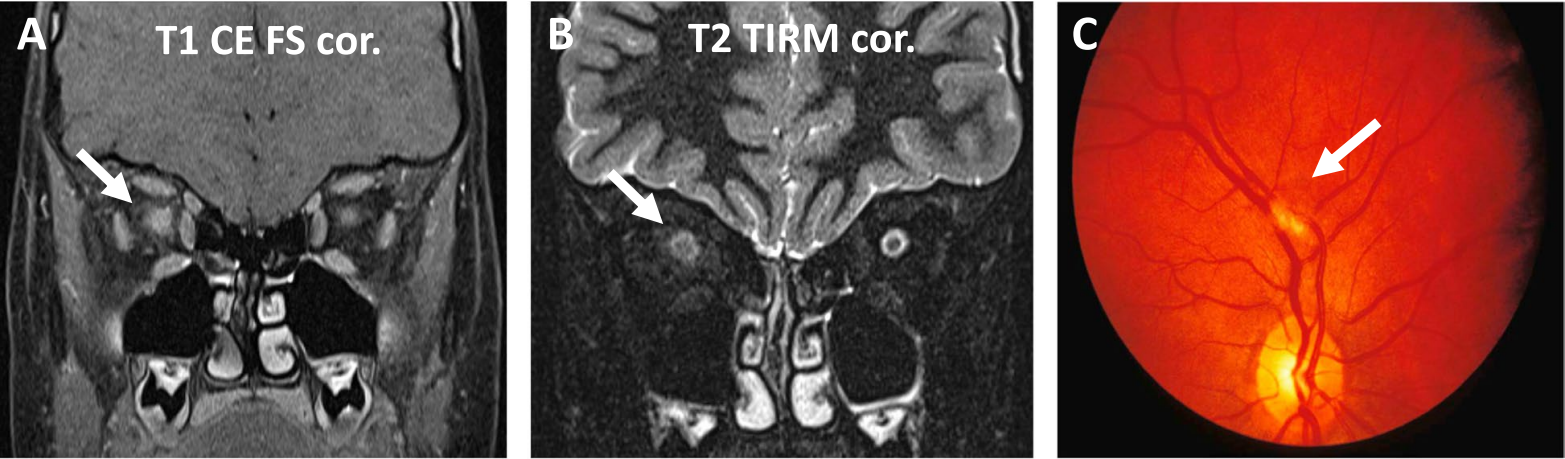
Neuromyelitis optica and retinitis in an 8-year-old jSLE patient. A Coronal MRI images showing perineural contrast enhancement of the right optic nerve in T1-weighted sequences (arrow). B Increased T2-weighted signal of the *Nervus opticus* and reduced signal of the nerve sheath (arrow) representing focal oedema of nerve and optic perineuritis. C Cotton-wool infiltrates resembling retinitis of the right eye in the same patient. The patient failed to respond to treatment with CPM and high-dose corticosteroids and was therefore treated with B cell depletion (rituximab), followed by repeated/cyclic immune-apheresis
